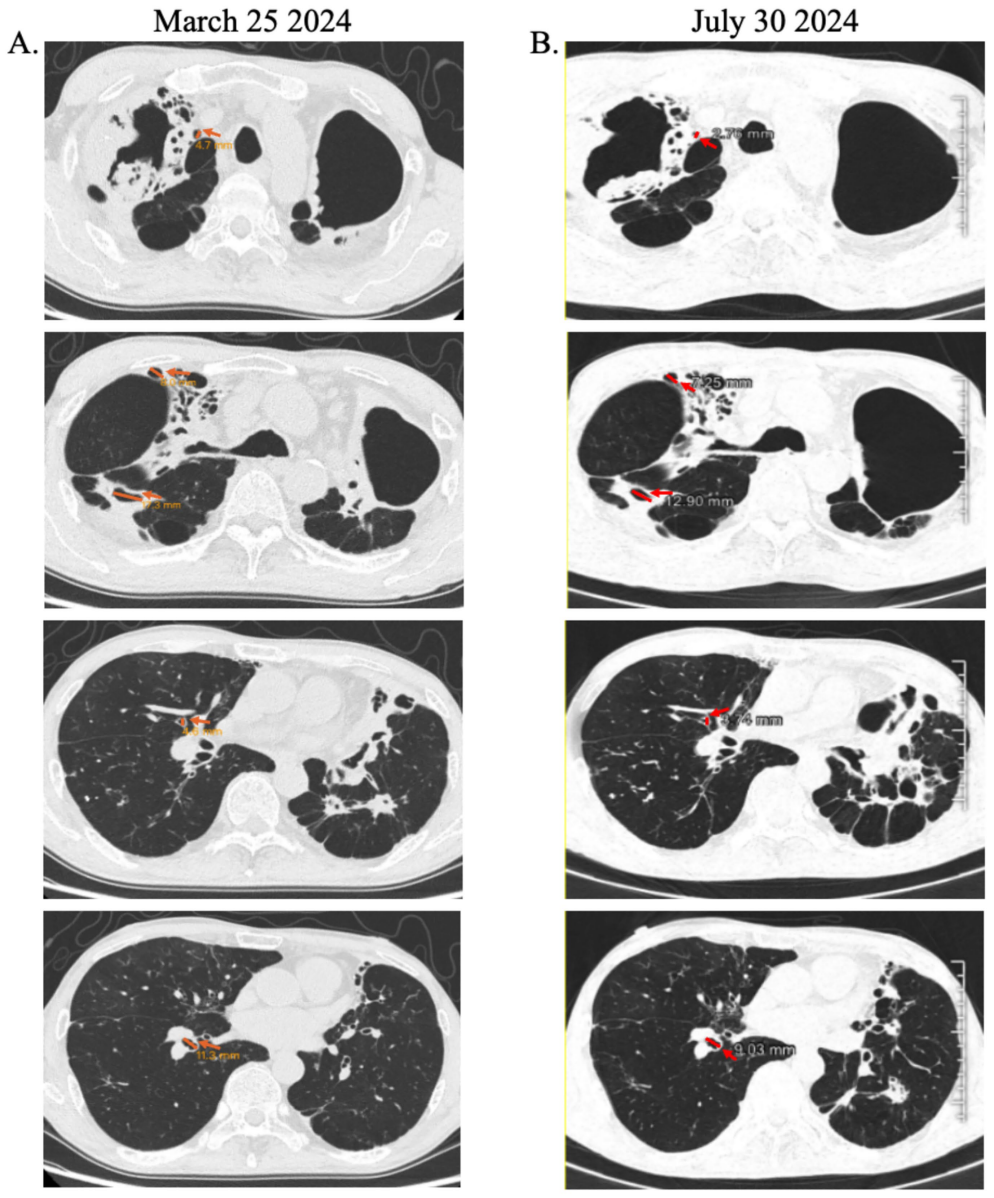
Radiographic evidence of air cavity reduction following HucMSC-Exos inhalation therapy. (A) Baseline chest CT scan demonstrating extensive cavitary lesions (yellow arrows), (B) follow-up scan showing significant reduction in cavity dimensions (red arrows).
